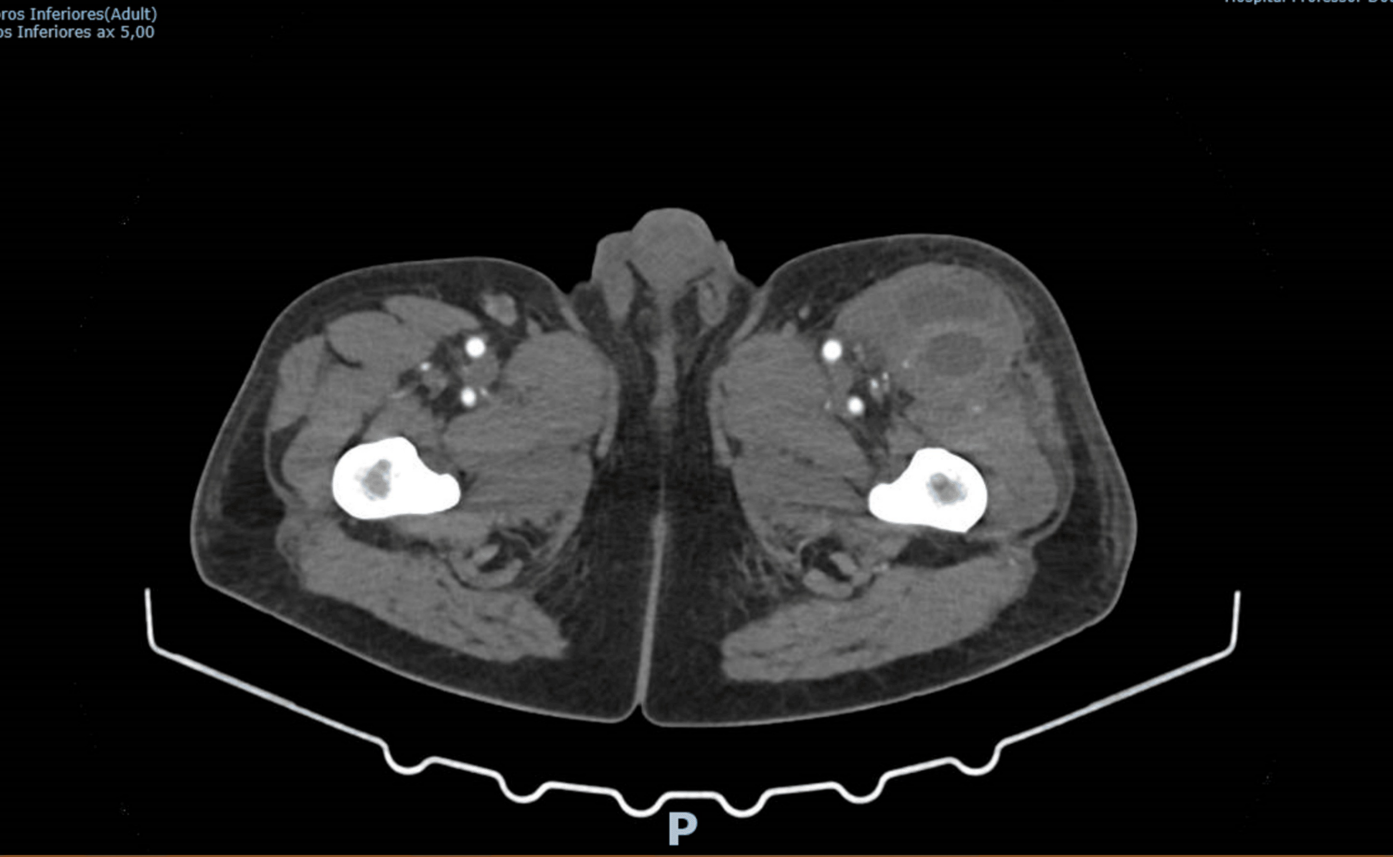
CT scan showing a multiloculated intramuscular collection on the anterolateral aspect of the mid-third of the right leg CT scan showing a multiloculated intramuscular collection on the anterolateral aspect of the mid-third of the right leg, measuring approximately 4.5 x 4.3 cm in its largest transverse dimensions and 13.7 cm in longitudinal length, also showing peripheral enhancement after intravenous contrast administration, suggestive of hematoma versus abscess, without active contrast extravasation.
